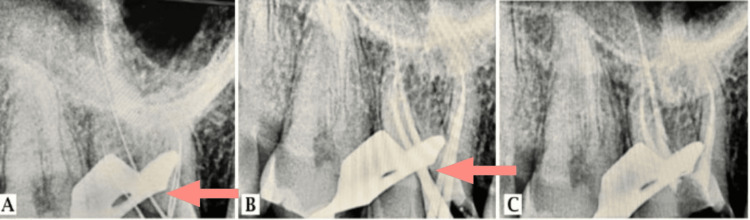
Radiographs of (A) working length, (B) master cone, and (C) obturation were taken using RVG RVG: radiovisiography
